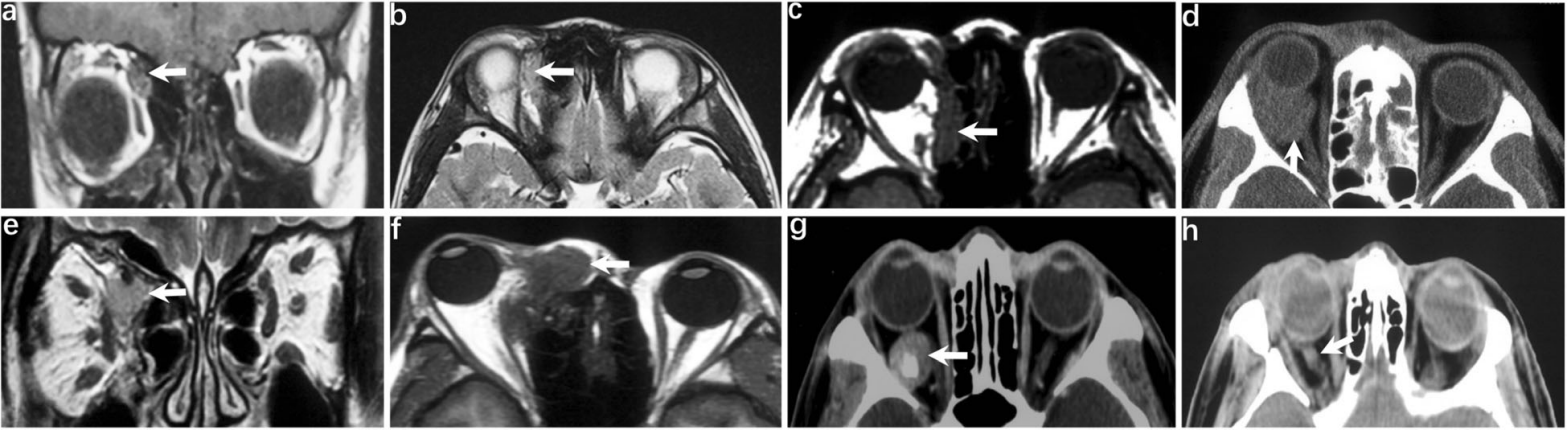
a and b MRI of case 1. a coronal T1WI showing the superonasal mass (arrow). b Axial T2WI showing an ill-defined and heterogeneous mass and adjacent medial rectus (arrow). c MRI of case 2. Axial T1 showing an ill-defined and heterogeneous superonasal mass and adjacent medial rectus (arrow). d Axial CT of case 3. A well-defined intraconal mass adjacent to the anterior optic nerve (arrow). e and f MRI of case 4. e Coronal T1 W1 showing the superonasal mass and no adjacent medial rectus (arrow). f Axial T1 W1 showing the ill-defined and heterogeneous superonasal mass (arrow). g and h: CT of case 5. g Axial CT showing a well-defined intraconal lesion with a calcified mass (arrow). h Optic nerve was compressed and dislocated but integrated into the structure (arrow)
